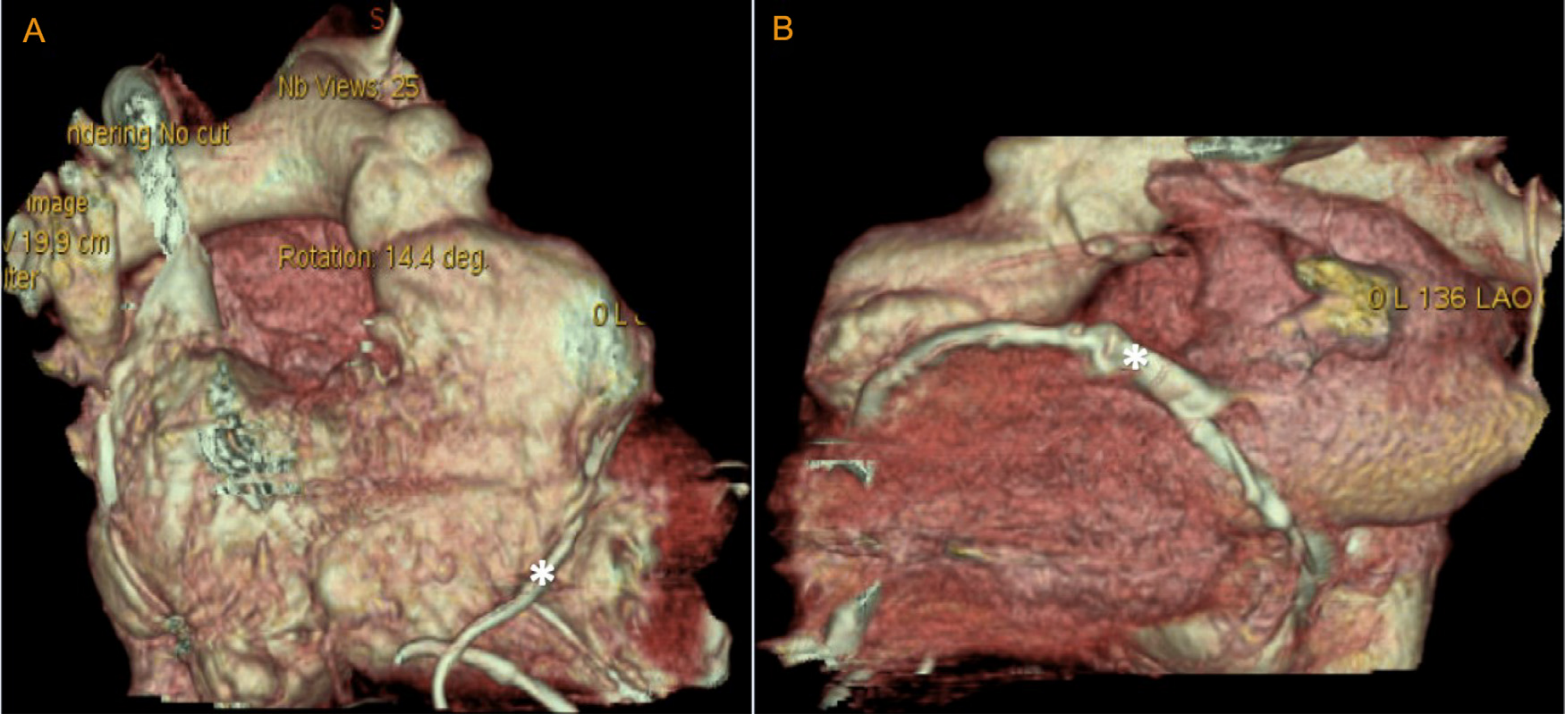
Three-dimensional computed tomography showing (A) epicardial pacemaker lead loop around the (A) anterior and (B) posterior surface of the heart (asterixis).
